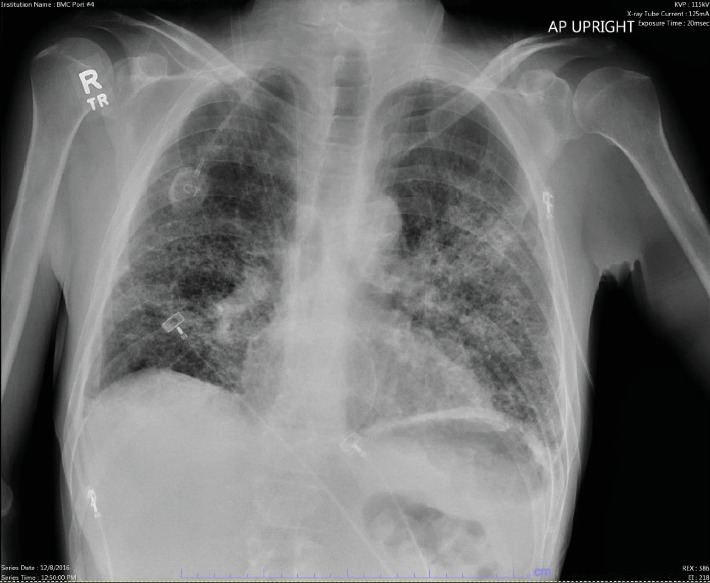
Chest radiograph showing decreased infiltrates after increased doses of steroids.
